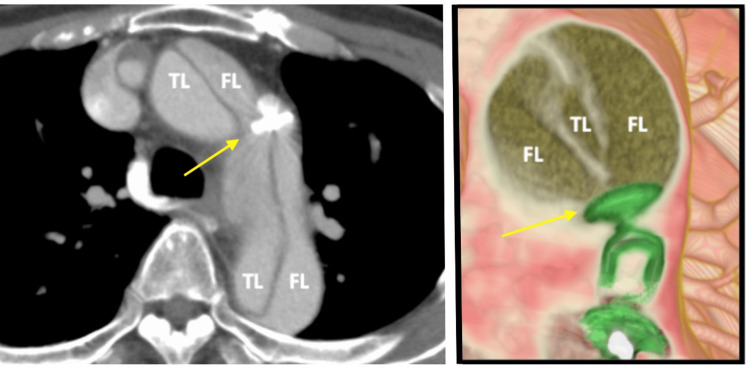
Intimal tear The following day after PDA closure, computed tomography scan revealed acute type A aortic dissection with intimal tear at the site of AVPII (yellow arrows). The device was migrated into the false lumen of the aorta. (Green device: AVPII) TL: True lumen; FL: False lumen; PDA: Patent ductus arteriosus; AVPII: Amplatzer Vascular Plug II
